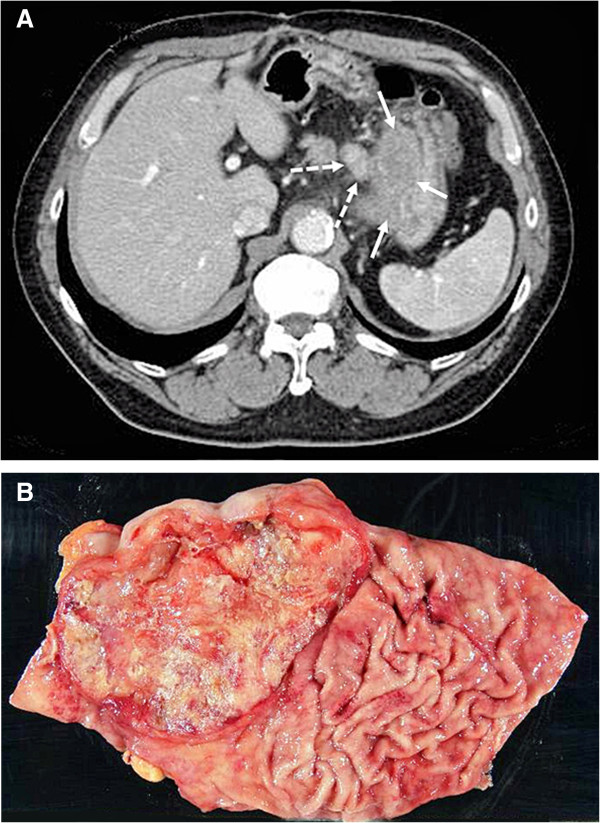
Tumor location and form Tumor location and form. Abdominal computed tomography (CT) scans showed carcinoma at the esophagogastric junction (white arrow) and lymph node swelling (dotted arrow) before surgery (A). Macroscopic findings of the resected stomach: a Borrmann type III tumor that measured approximately 100 mm in diameter was located around the esophagogastric junction (B).
